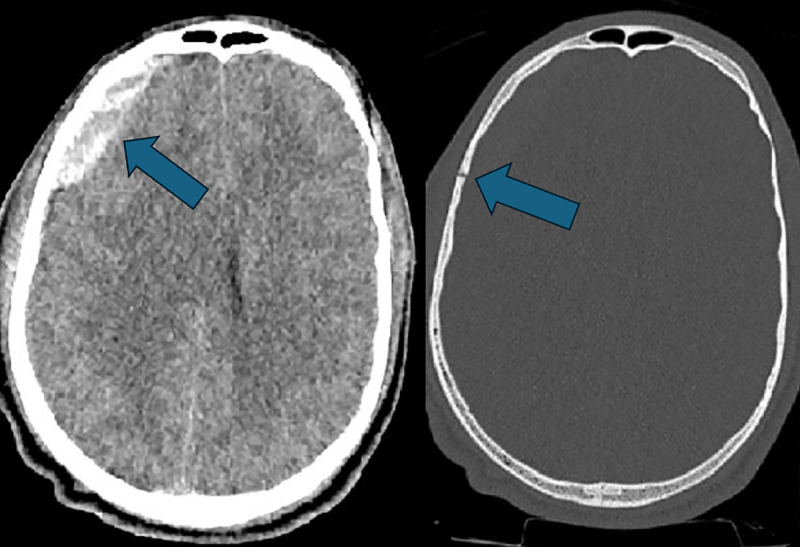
Head CT: skull fracture with underlying epidural hematoma.
